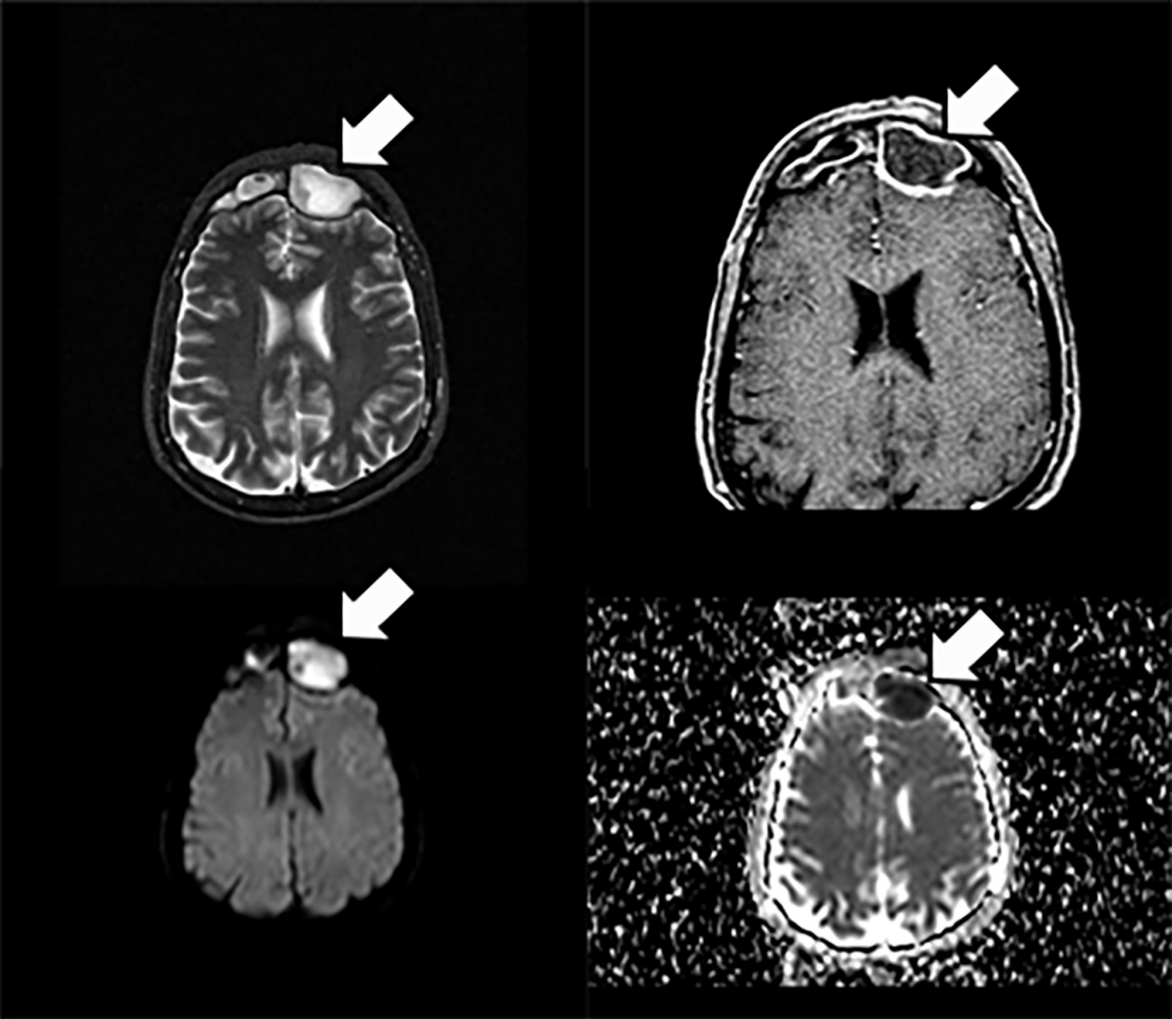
MRI scan (Case 1) Magnetic resonance imaging (MRI) showed enhancing lesion filling the left frontal sinus and erosion of the internal wall of the frontal sinus with extension to the anterior cranial fossa. Short tau inversion recovery (STIR), diffusion-weighted imaging (DWI), and apparent diffusion coefficient (ADC) showing diffusion restriction as well as enhancement and thickening of dura mater (white arrows).
